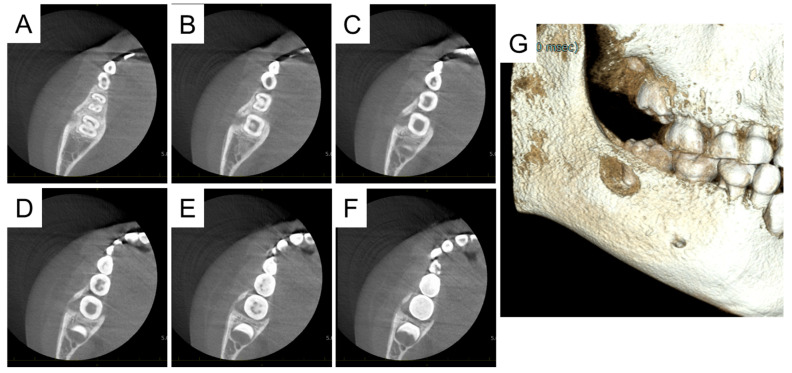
CBCT revealed an alveolar bone defect around the right mandibular first molar, confirming the diagnosis of a dentoalveolar abscess (A–G). In cooperation with the oral surgery department, surgical procedures were performed the next day. The gingival sulcus was incised, and the thinned alveolar bone and surrounding granulation tissue were removed. Antibiotic-containing gauze was placed, and the wound was sutured. Antibiotics and painkillers were prescribed, and the gauze was removed 3 days later. The pain was under control without the use of painkillers, and the redness, induration, and swelling were showing signs of amelioration. No purulent discharge was observed from the wound.
